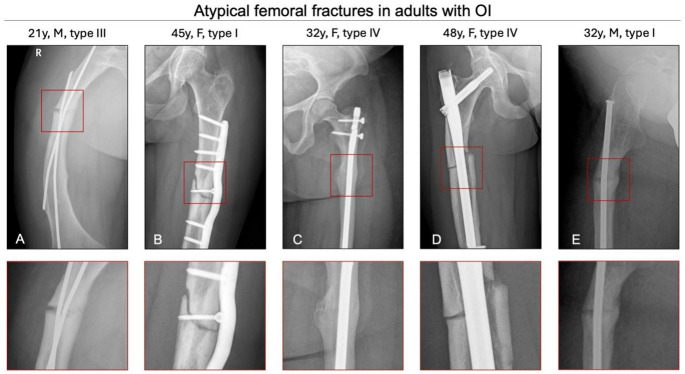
X-ray images of individual adult patients with classical OI who had an atypical femoral fracture (AFF). Above each individual X-ray, the corresponding age in years, sex (F: female; M: male), and OI type are indicated
